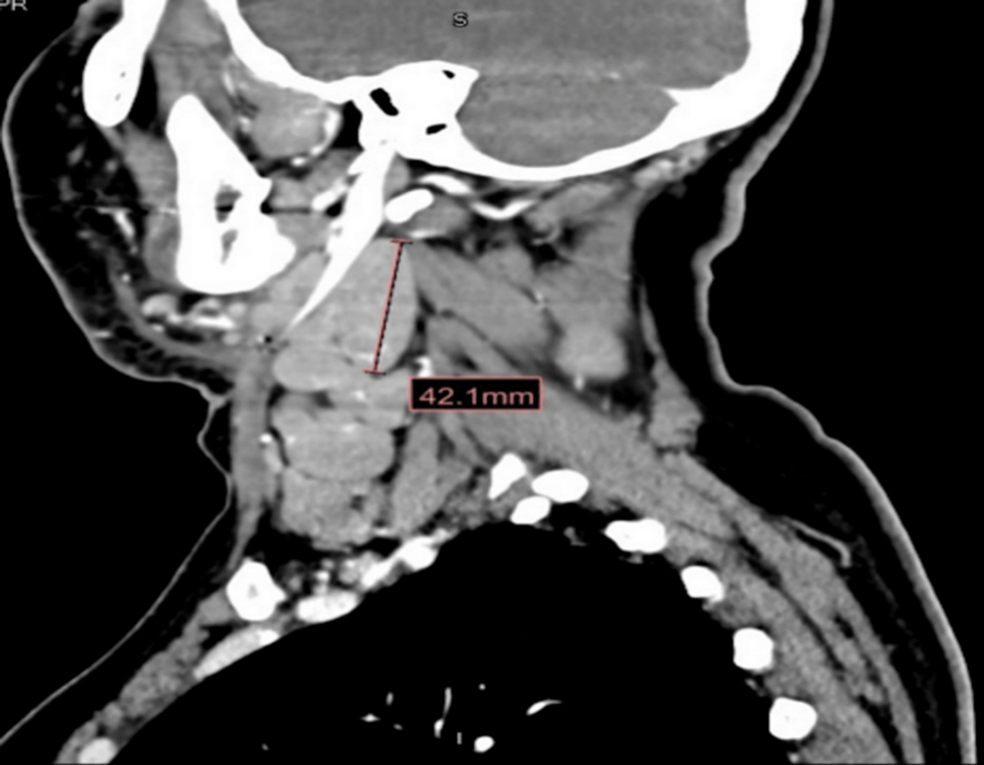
CT neck with contrast (coronal view) showing severe bilateral neck lymphadenopathy most prominent on the right side measuring up to 4.2 cm at the jugulodigastric region level (2A and 2B), uplifting the right sternocleidomastoid muscle CT, computerized tomography
